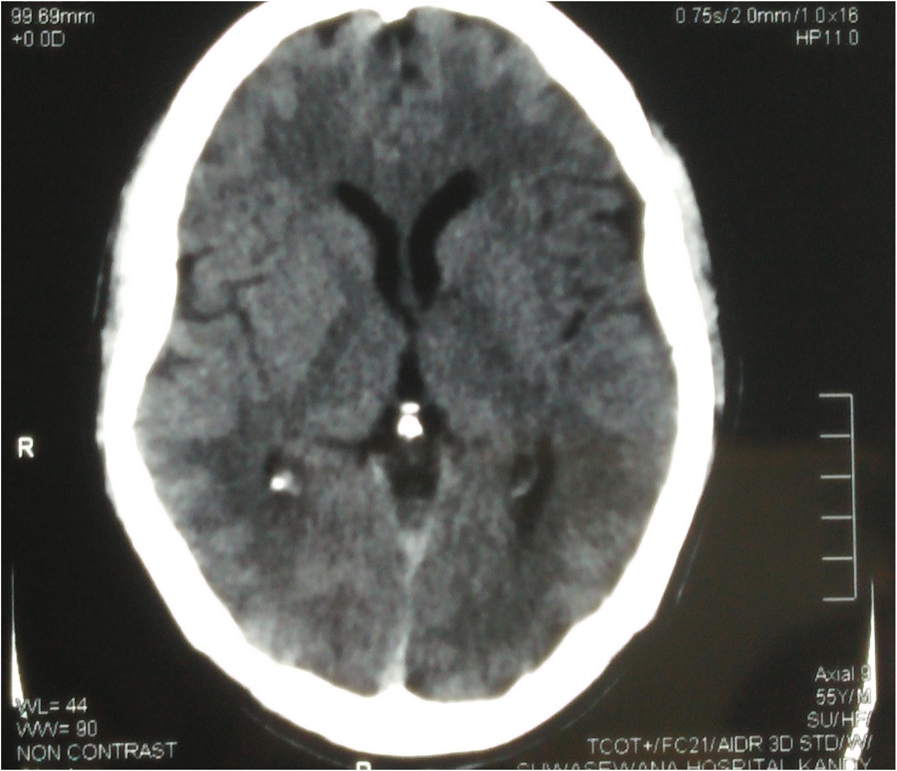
Non contrast computer tomography (CT) of the brain showing prominent cerebral white matter with obliterated sulci
